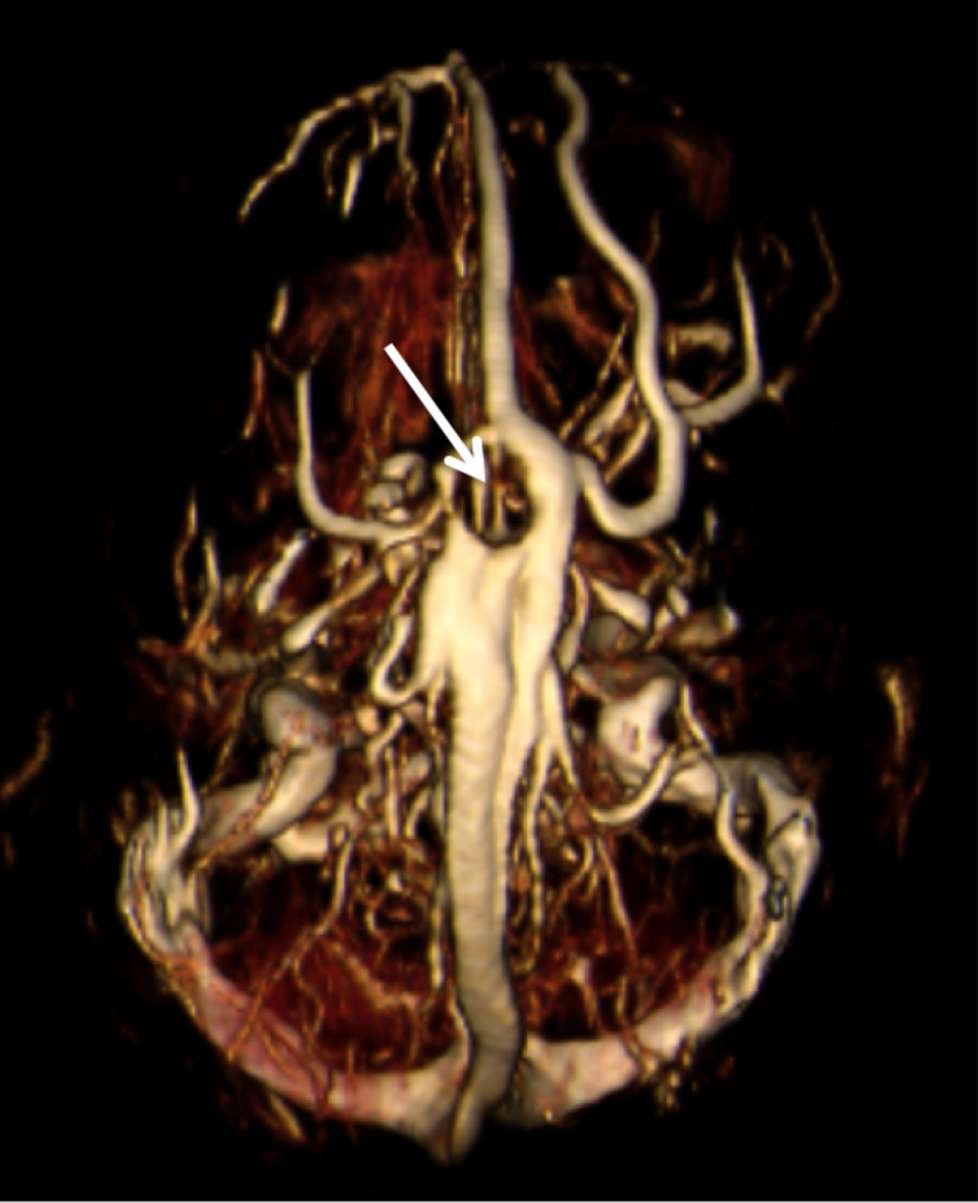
T2-weighted coronal MRI of the brain noting giant AG (arrow).
